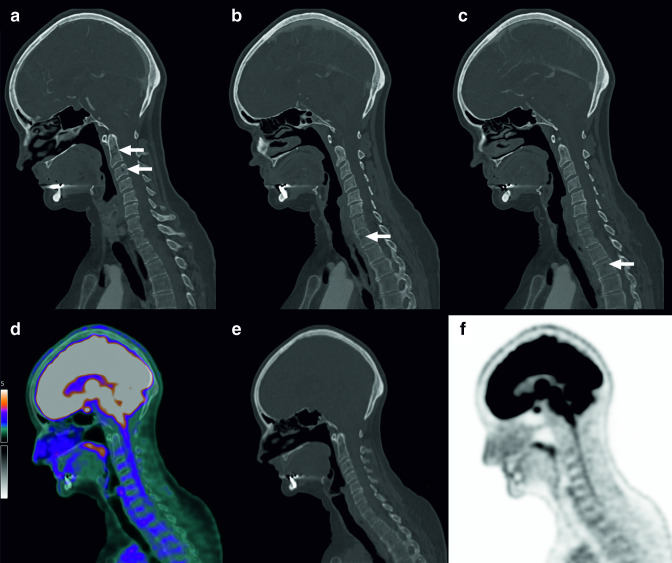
A 62-year-old female presented with suspected acute ischemic stroke, for which CTA of the head and neck was ordered to identify large vessel occlusions suitable for endovascular thrombectomy. CTA angiography was negative for large vessel occlusions, but did show a few hypodense foci of unclear nature in vertebrae C2, C3, T1, and T3 (a–c, arrows) as incidental findings. FDG PET/CT was ordered to evaluate the spine for metastatic disease and to search for a primary tumor. However, FDG-PET/CT did not show any pathology (FDG-PET/CT (d), low-dose CT (e), and FDG-PET (f) images at the level of the cervical—upper thoracic spine are shown). Follow-up MRI 6 months later did not show any pathology either. Therefore, the lesions were classified as benign. CTA, CT angiography; FDG, ^18^F-fluoro-2-deoxy-D-glucose; PET, positron emission tomography.
